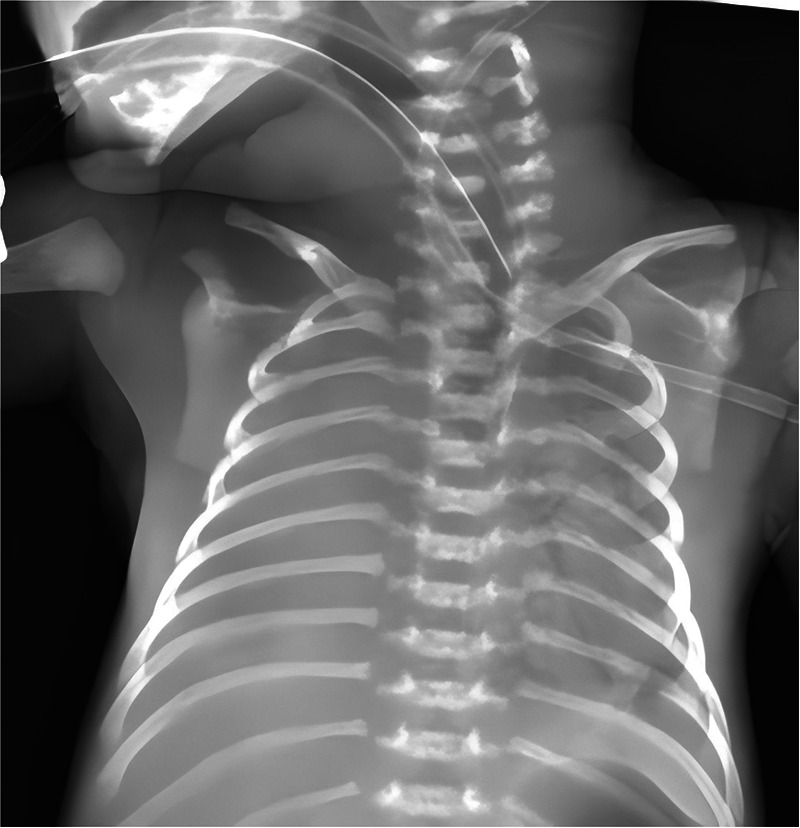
Bedside chest x-ray during resuscitation showing neonatal respiratory distress syndrome with diffuse ground-glass opacification and air bronchograms, more pronounced on the right.
